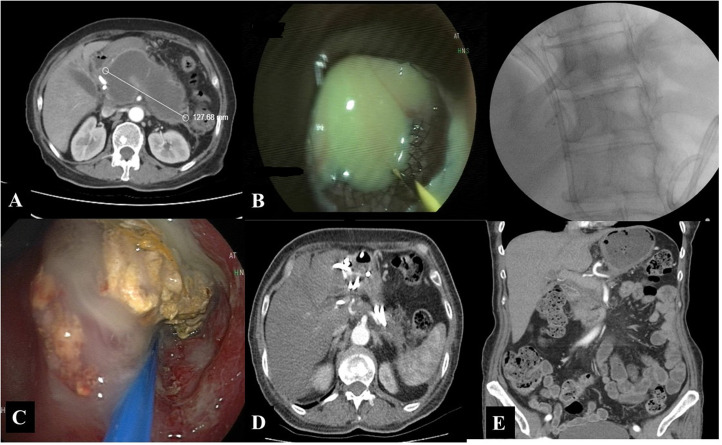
A 68-year-old female patient. A) A 12 cm-sized WON along the pancreas. B) Placement of a LAMS and a nasocystic catheter into the WON cavity using an endoscopic approach. C) Endoscopic necrosectomy. D) Follow-up image showing pigtail pigtail plastic stents 2 months post-procedure. E) Complete resolution of WON seen on a follow-up CT scan 4 months after the procedure.
